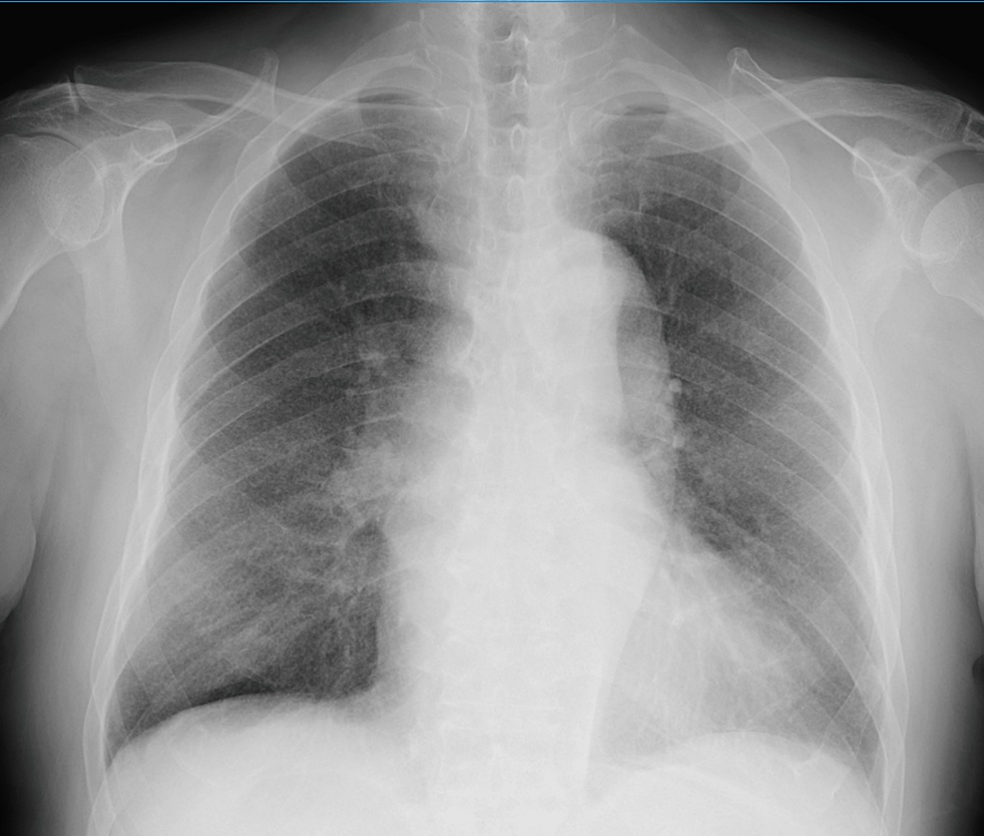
Chest X-ray Chest X-ray showing diffuse bilateral miliary nodules.
